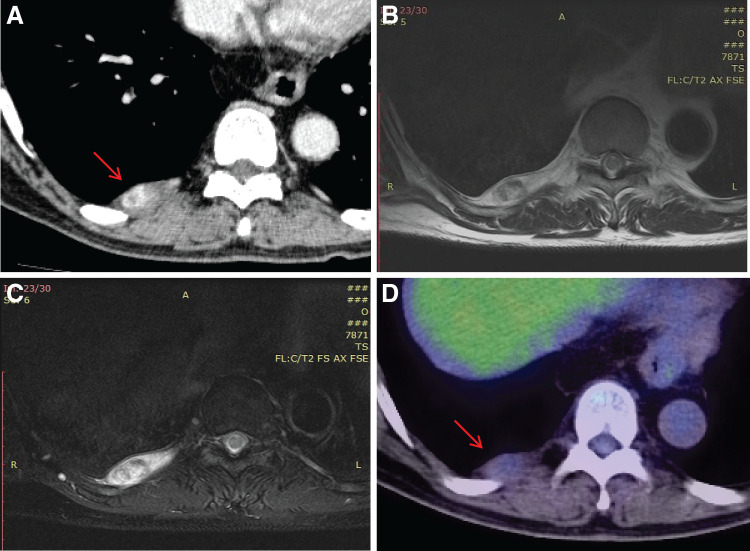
Radiological findings of the chest wall tumor. (A) Contrast-enhanced CT scan showing a well-circumscribed, heterogeneously enhanced oval mass in the right posterior chest wall at the level of the ninth rib (arrow). (B) T2-weighted MRI revealing heterogeneous high signal intensity in the tumor. (C) Fat-suppressed T2-weighted MRI clearly delineating the tumor from the surrounding fatty tissue. (D) FDG-PET/CT fusion image showing mild accumulation in the tumor with a SUVmax of 2.2 (arrow). FDG-PET/CT ^18^F-fluorodeoxyglucose PET/CT; SUVmax, maximum standardized uptake value
